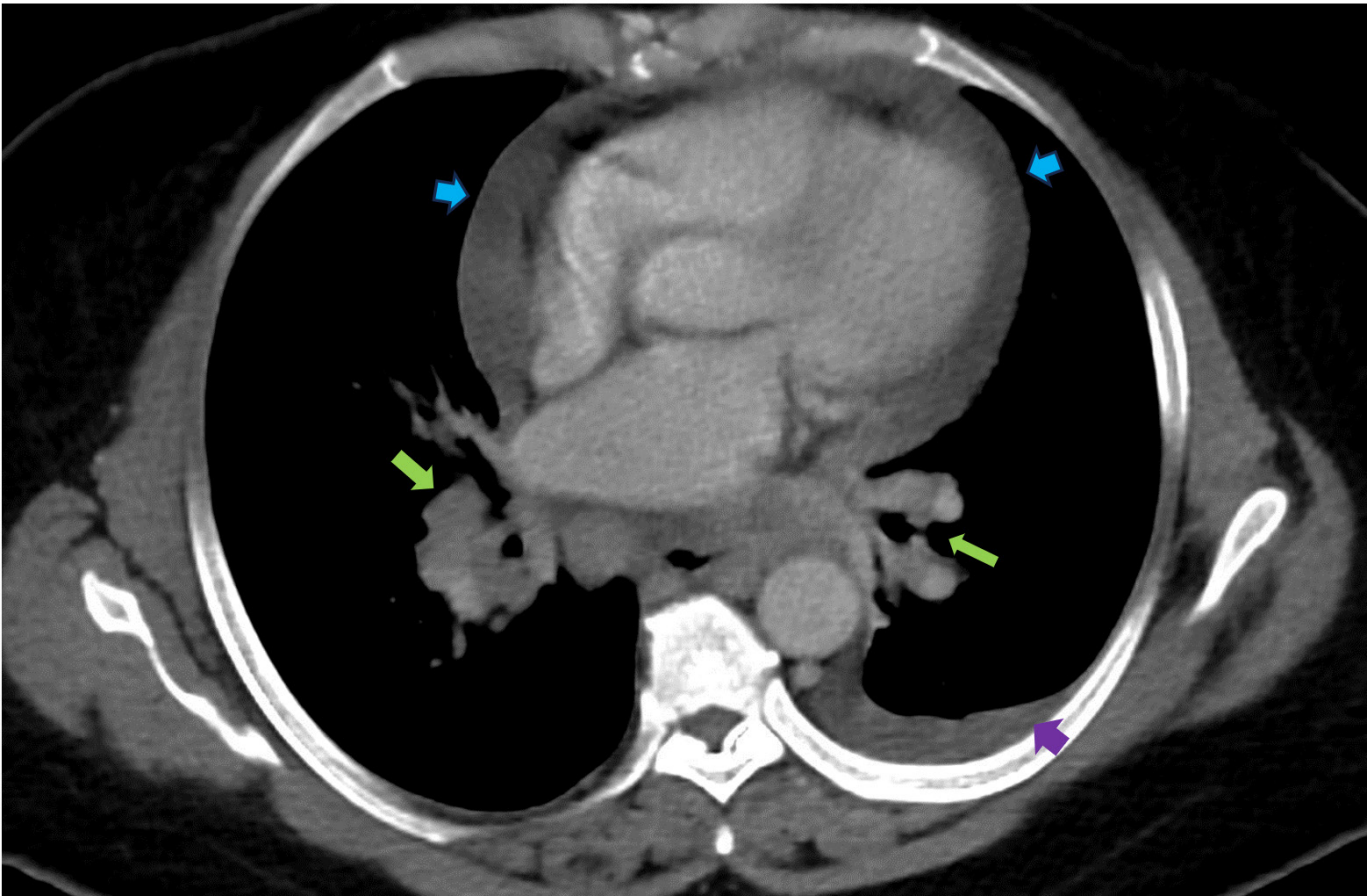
CTA of the chest: Blue arrows indicate a moderate‐size pericardial effusion. The purple arrows highlight the left‐sided pleural effusion. The green arrows show evidence of bulky hilar lymphadenopathy bilaterally.
